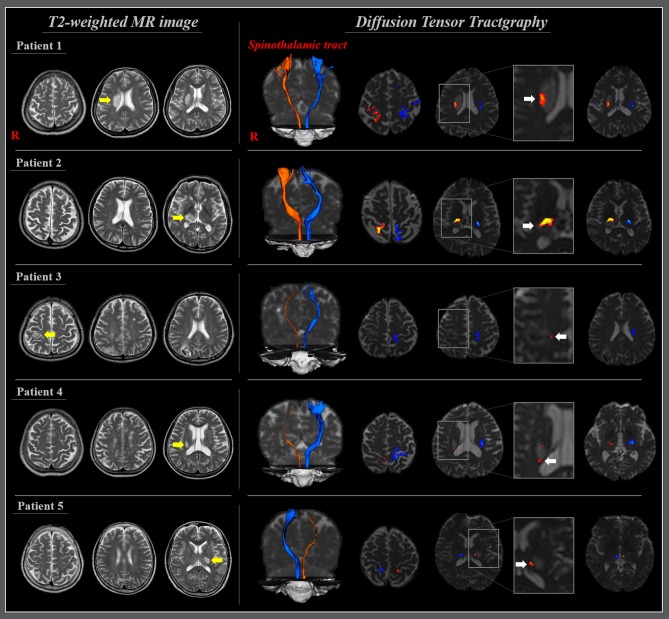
T2-weighted MRI and diffusion tensor tractography of patients with CPSP following cerebral infarction. T2-weighted MR images of five patients with cerebral infarction (yellow arrows). Diffusion tensor tractography of five patients at 11 days on average after stroke onset; all the reconstructed spinothalamic tracts in the affected hemisphere originated from the posterolateral medulla and terminated at the primary somatosensory cortex through adjacent part of the infarct (white arrows) and narrowing in three patients (patients 3, 4, and 5) [reprinted with permission from Jang et al. (30)].
